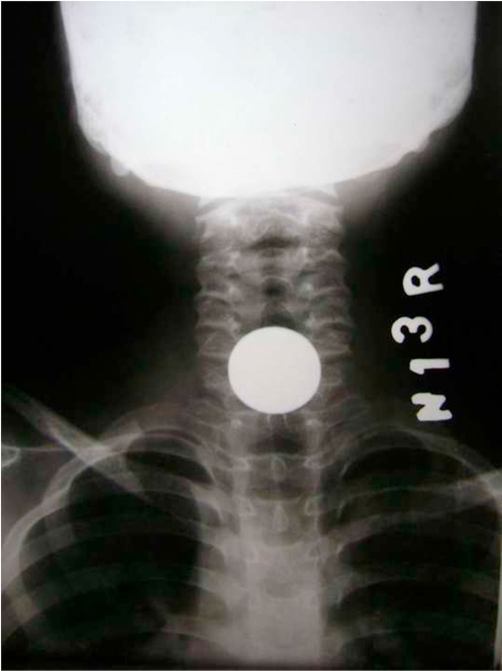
Lateral view X-ray showing double coin in upper esophagus.
